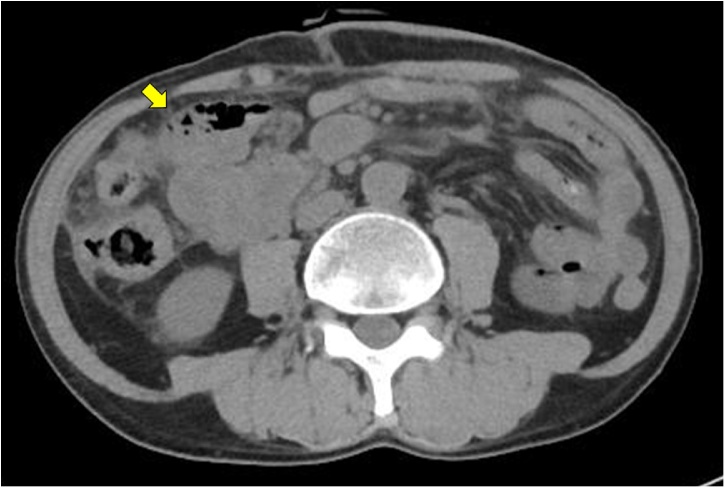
Abdominal CT after 6 courses of neoadjuvant chemotherapy. After 6 courses of treatment, the primary tumor has shrunk remarkably to 5.0 cm. Invasion to surrounding organs is not observed.
